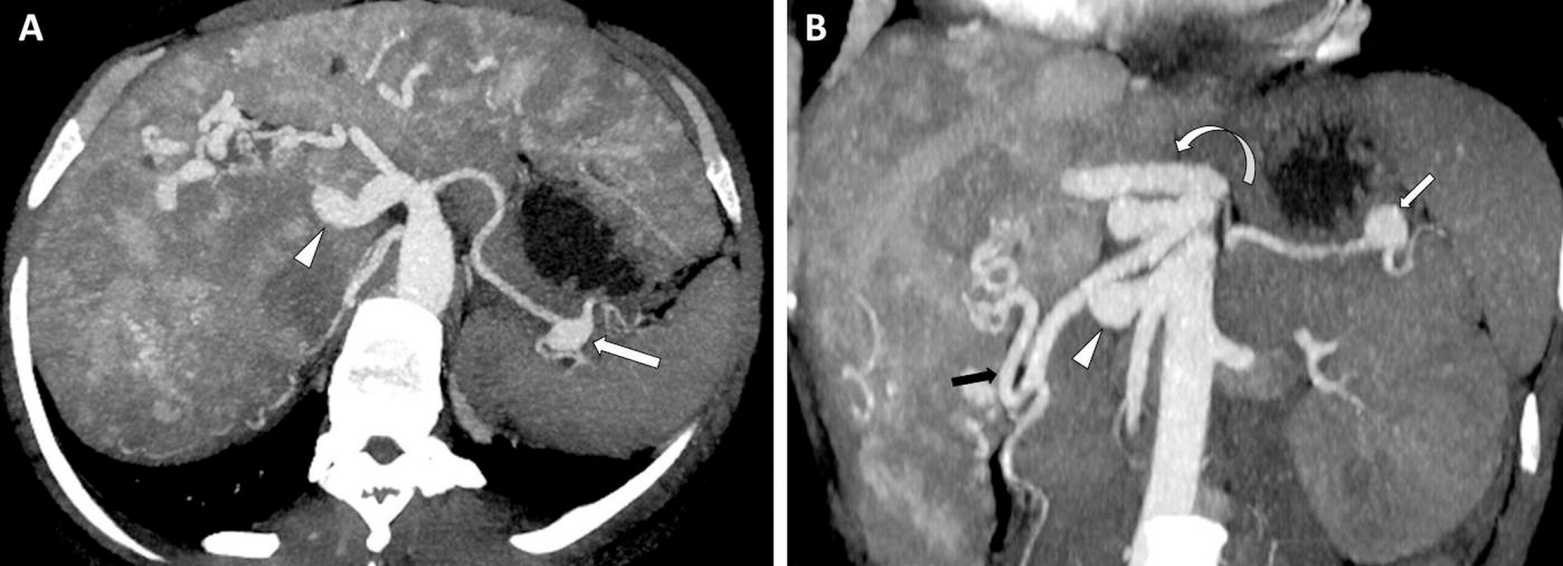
SAA in a 50 year-old woman with HHT and severe liver involvement. Enhanced computed tomography in oblique axial (A) and oblique coronal (B) Maximal Intensity Projections (12mm thickness). A. a 12x17mm fusiform hilar SAA is supplied by a 4mm splenic artery (arrow). The severe liver involvement is characterized by enlargement of arteries supplying the liver (right hepatic artery, 15.9 mm: arrowhead), by presence of arterio-portal and arterio-hepatic shunts and by hepatomegaly with heterogeneous enhancement. B. Three enlarged hepatic arteries supply the liver: A 4.5mm middle hepatic artery originating from the coeliac trunk (thin arrow). A 8mm left hepatic artery originating from the left gastric artery (curved arrow). A 12 mm right artery originating from the superior mesenteric artery (arrow). The increased hepatic blood flow contrasts with the splenic blood flow, which seems to be decreased by a probable steal phenomenon. Then, the SAA (star) has developed on a systemic artery, in spite of a decreased blood flow induced by the severe hepatic involvement. This reinforces the hypothesis of a vasculopathy at the origin of SAA.
